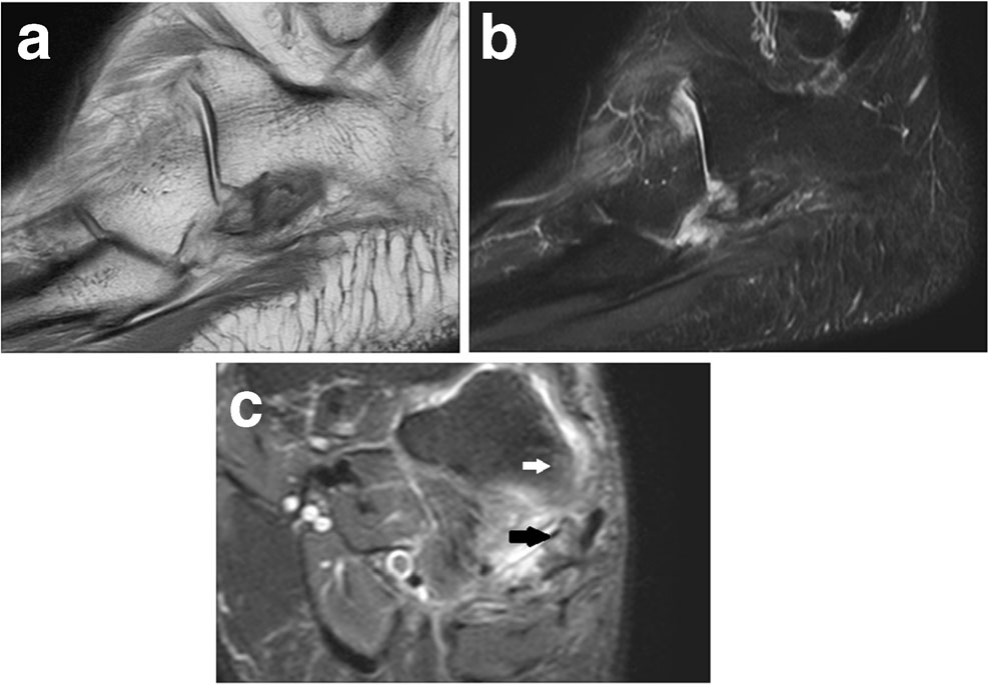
POPS – magnetic resonance imaging. *9A* T1w image, sagittal plane. *9B* T2w fat suppression image, sagittal plane. *9C* T2w fat suppression, coronal plane. MRI shows oedema of the bone marrow of the OP (*black arrow*) and cuboid (*white arrow*) as well as inflammatory changes in soft tissues
